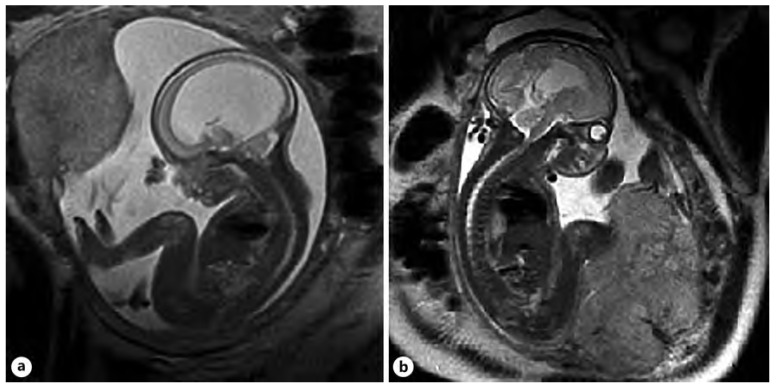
A comparison of the fetal MRI is shown in the sagittal plane, depicting the change in the lateral ventricular size of the brain pre- (a) versus post-VAVI (b) [127].
